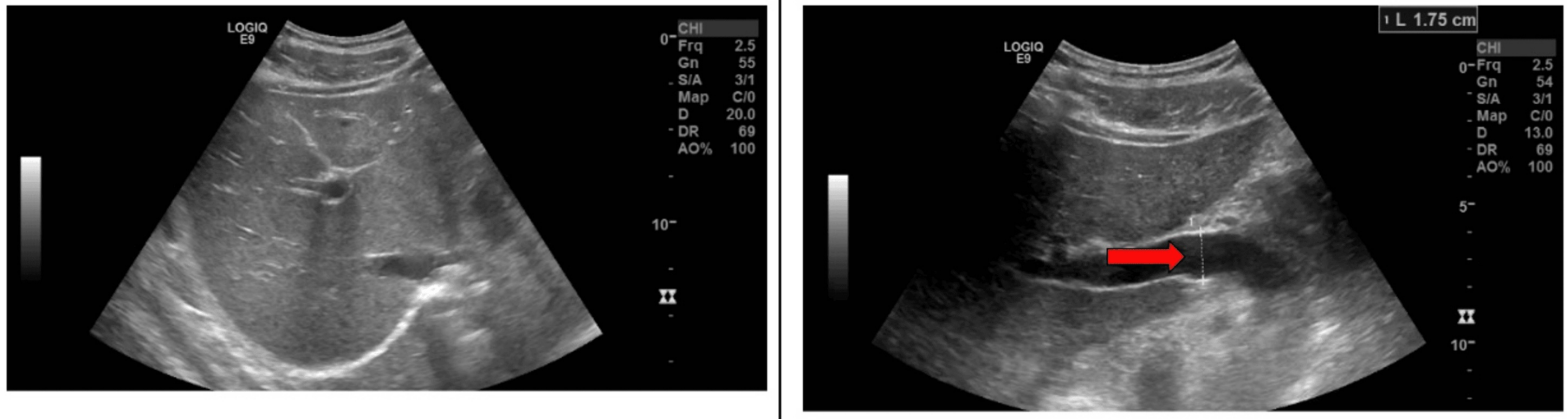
Hepatic Ultrasound (US) Left: US reveals heterogeneous hepatic architecture, no mass, normal gallbladder, and normal common bile duct. Right: US reveals mild dilation of the portal vein at 1.5 cm. No portal vein thrombosis seen.
